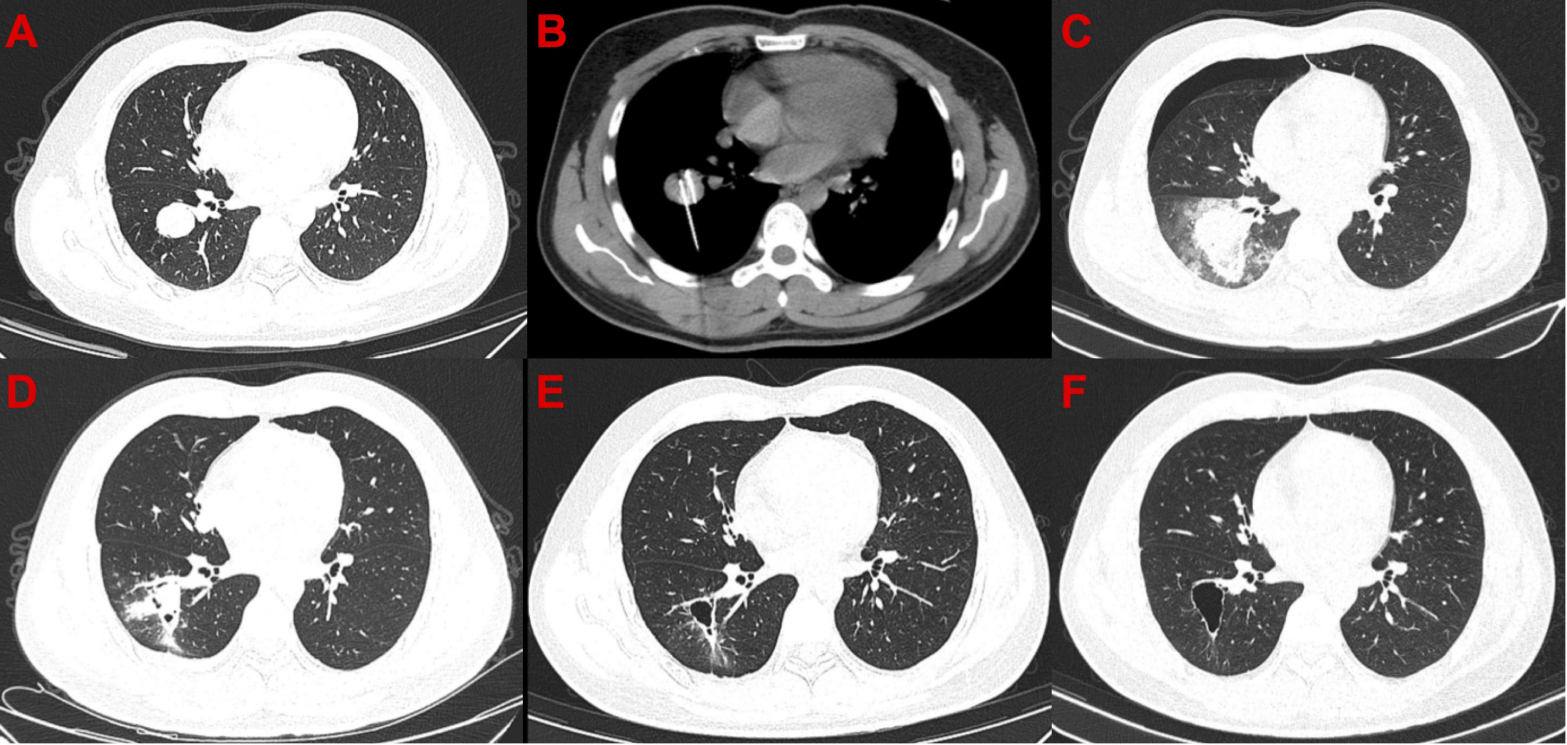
Computed tomography images of the only patient with complete response after cryoablation. (A) Before cryoablation. (B) During cryoablation. (C) After cryoablation, local large-scale inflammation and pneumothorax can be observed. (D) One month after cryoablation, pneumothorax disappeared, and the scope of inflammation narrowed. (E) Moreover, 3.5 months after cryoablation, cavity formation was observed in the diseased area. (F) 7.5 months after cryoablation.
